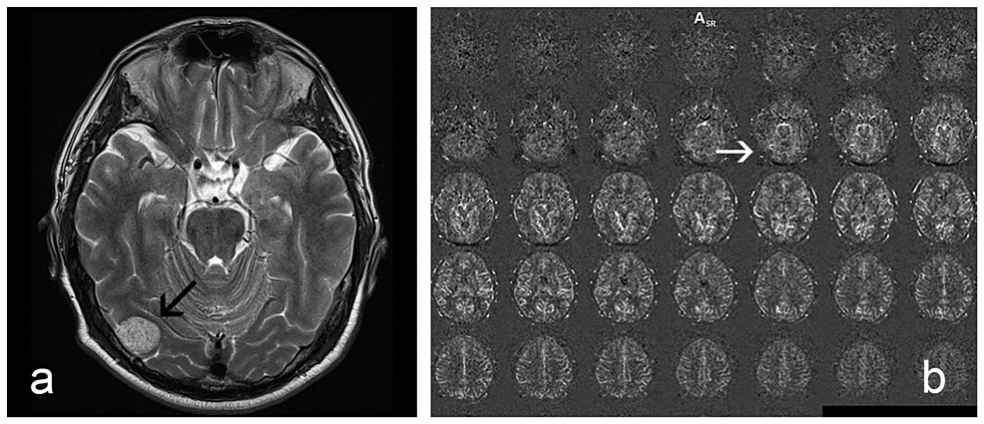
Meningioma in the right occipital region. (a) T2-weighted image displaying the tumor (black arrow). (b) Corresponding ASL images reveal no signal enhancement in the tumor area (white arrow).
